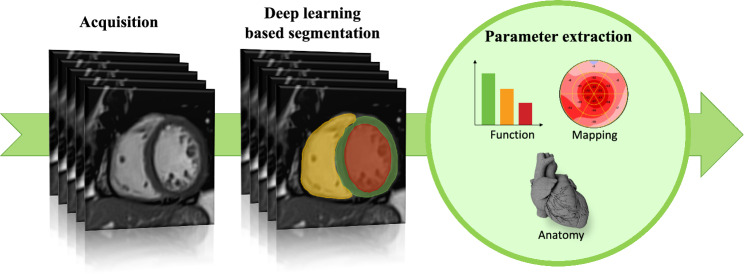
Example of different steps needed in parameter extraction for cardiac magnetic resonance imaging. From left to right: cardiac images are acquired, followed by automated segmentation of various anatomical heart structures, enabling subsequent parameter extraction
